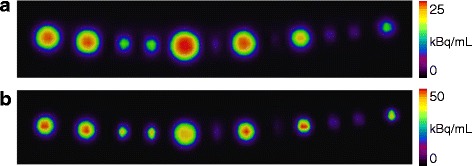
The spheres in air (diameters in mm: 10, 10, 6, 6, 12, 4, 10, 3, 8, 4, 4, and 6) scanned on a Siemens Biograph 64 Truepoint PET/CT. Two reconstructions of the same PET data are shown. a Without PSF (Iterative3D, 6 iterations, 21 subsets, 2-mm Gaussian filter, 336 × 336 matrix) and b With PSF (TrueX using same reconstruction parameters including PSF). Note that different intensity scales were used on the two images to allow visualization of all spheres (RCs are higher with PSF). Without PSF, the well-known pattern is seen: the RCs drop monotonically as function of sphere diameter. With PSF, the rank order of RCs is: 8, 10, 12, 6, 4, and 3 mm
